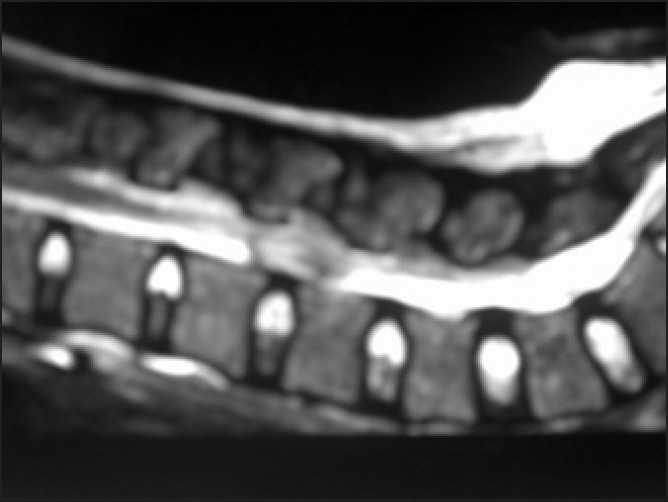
MRI of the spinal cord showing arachnoiditis
